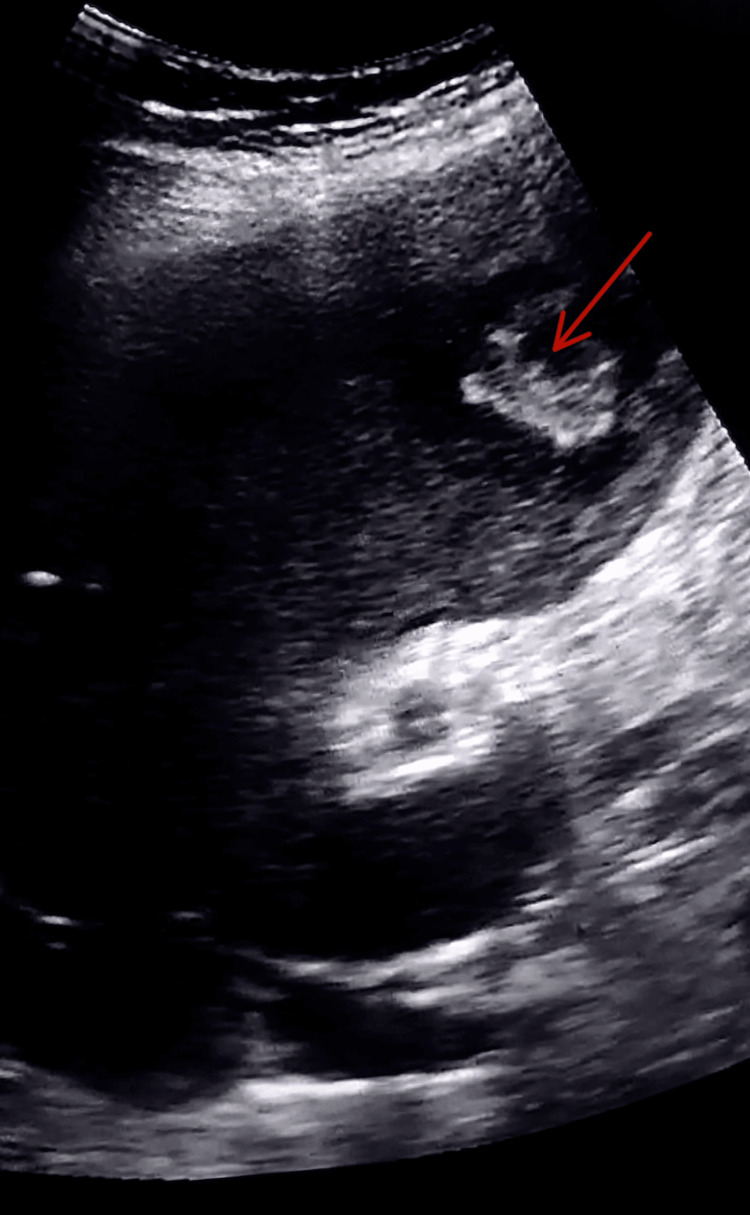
Ultrasonography of the abdomen showing multiple hyperechoic lesions in both lobes of the liver with hepatomegaly suggestive of abscess-like lesions
